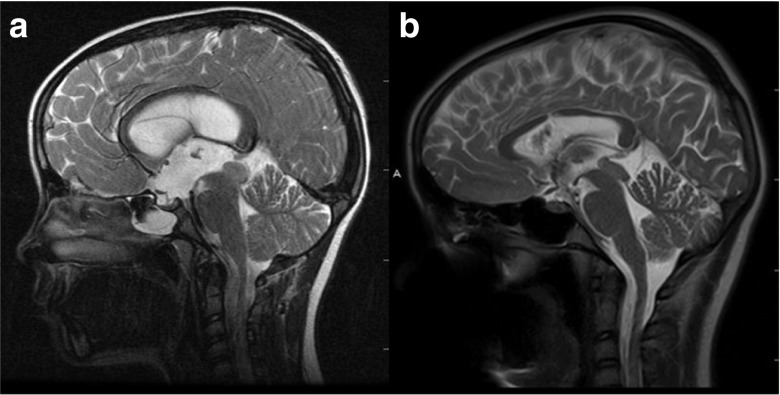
An 8-year-old female presented with headaches and papilledema. Preoperative T2-weighted MR (a) shows a tectal plate lesion and obstructive hydrocephalus. T2-weighted MR (b), 7 years after ETV, shows a reduction of the ventricles with flow void at the floor of the third ventricle
